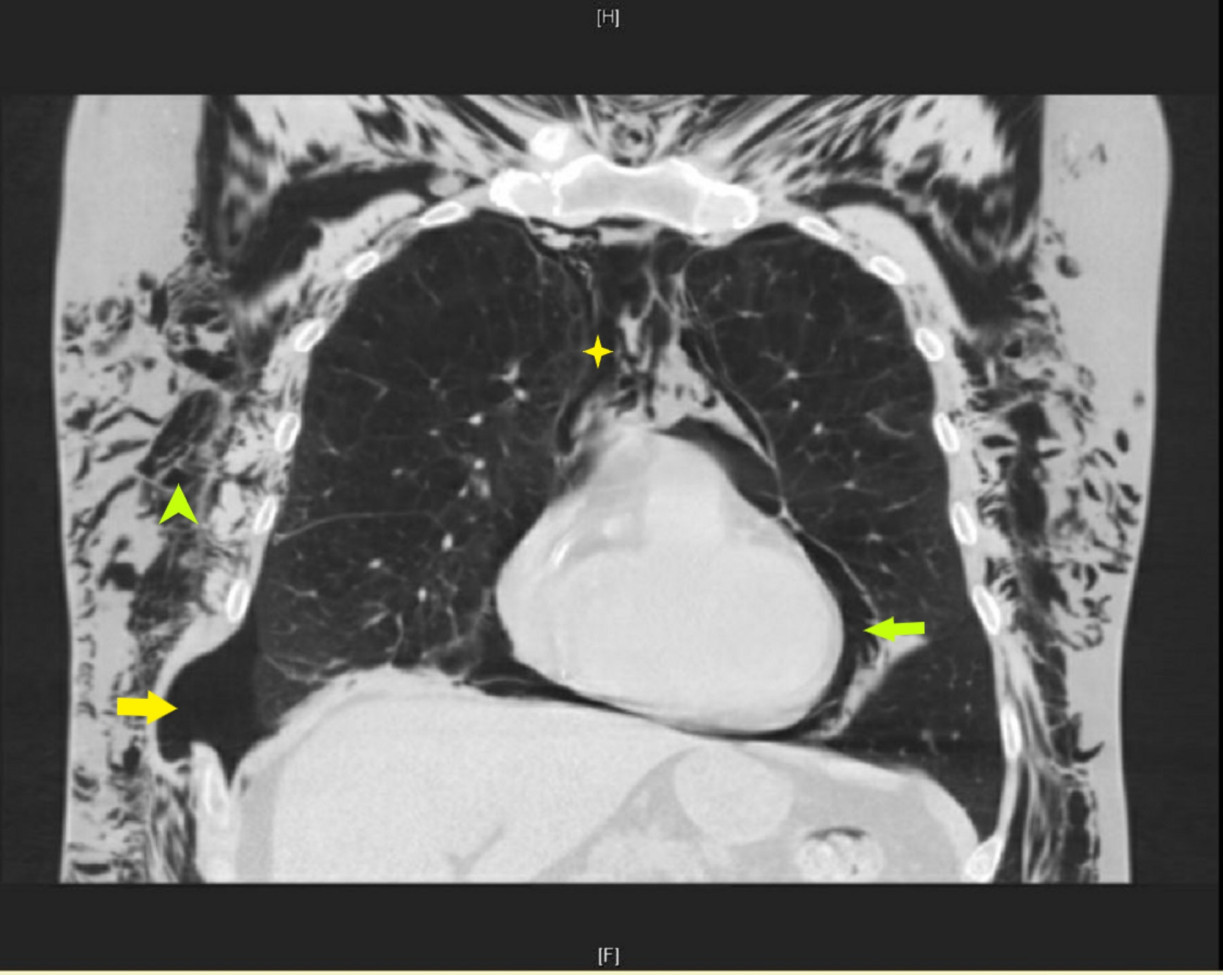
CT chest coronal section showing right-sided pneumothorax (yellow arrow) at the site of the previous herniation with extensive subcutaneous emphysema (green arrowhead), pneumomediastinum (yellow star), and pneumopericardium (green arrow) CT: computed tomography
